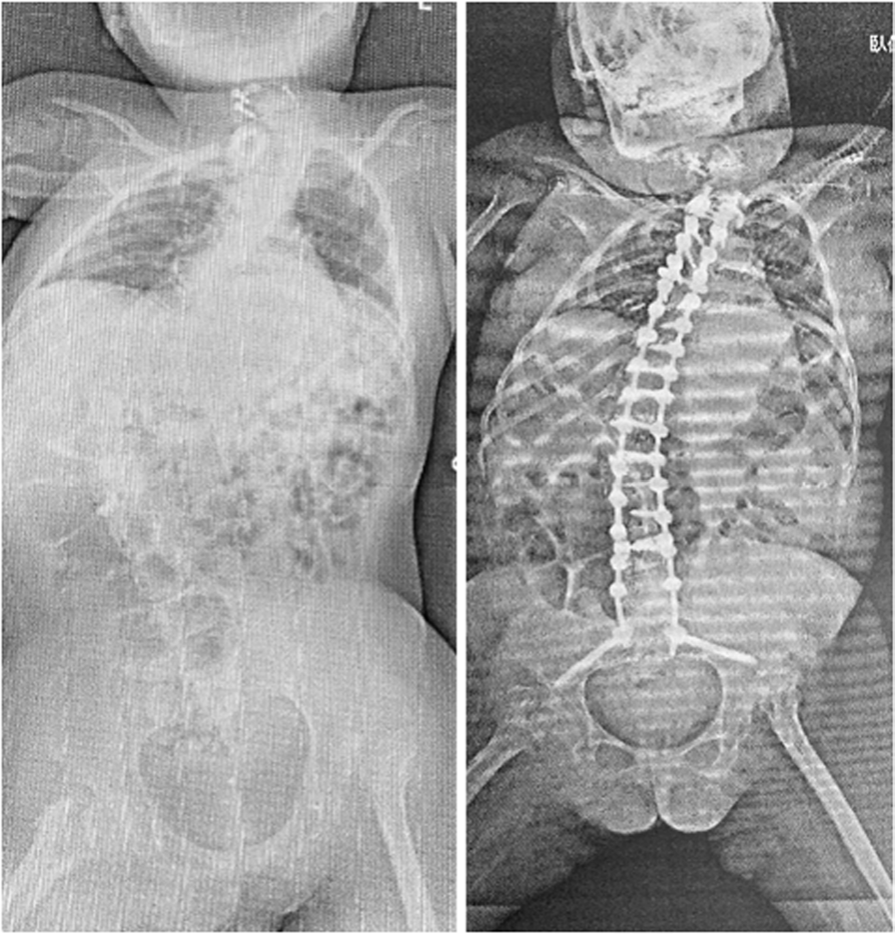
Pre- and postoperative X-rays of posterior spinal instrumentation and fusion (PSIF) for scoliosis
